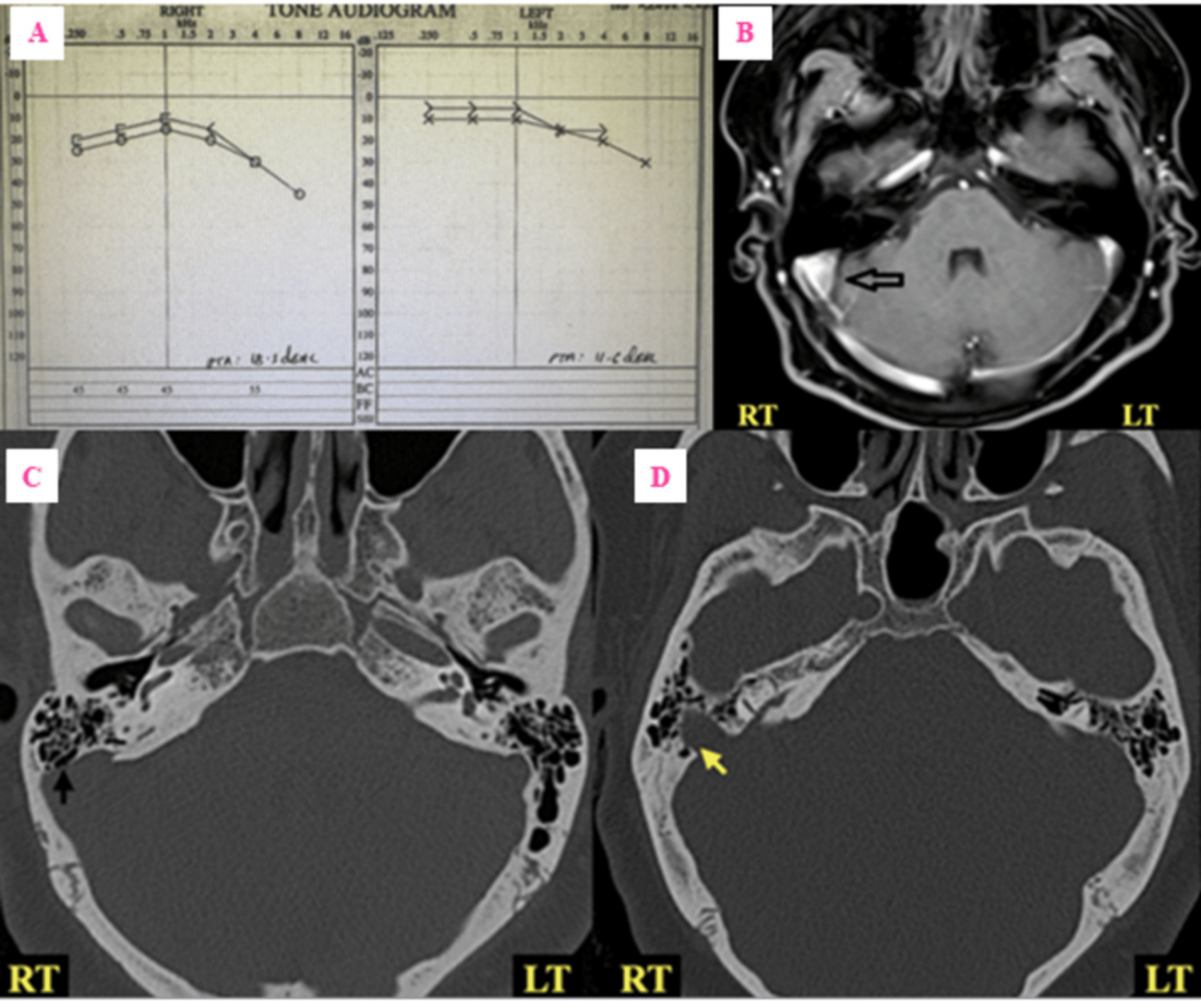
(A) Pure tone audiogram of right and left ears, (B) MRI, and (C, D) CT displaying right-sided unilateral sigmoid sinus diverticulum and sinus plate dehiscence (A) Pure-tone audiogram showing moderate sensorineural hearing loss involving both low and high frequencies on the right side and minimal high-frequency sensorineural hearing loss on the left side. (B) Contrast-enhanced T1 fat-suppressed image in axial plane displaying right-sided sigmoid sinus diverticulum (arrow) with focal protrusion of sigmoid sinus towards the mastoid air cells. (C) Absence of normal bony coverage (arrow) of the right sigmoid sinus with air-on-sinus sign. There is a normal bony covering over the left sigmoid sinus. (D) Axial high-resolution CT (HRCT) image depicting the right-sided sigmoid sinus diverticulum (arrow).
